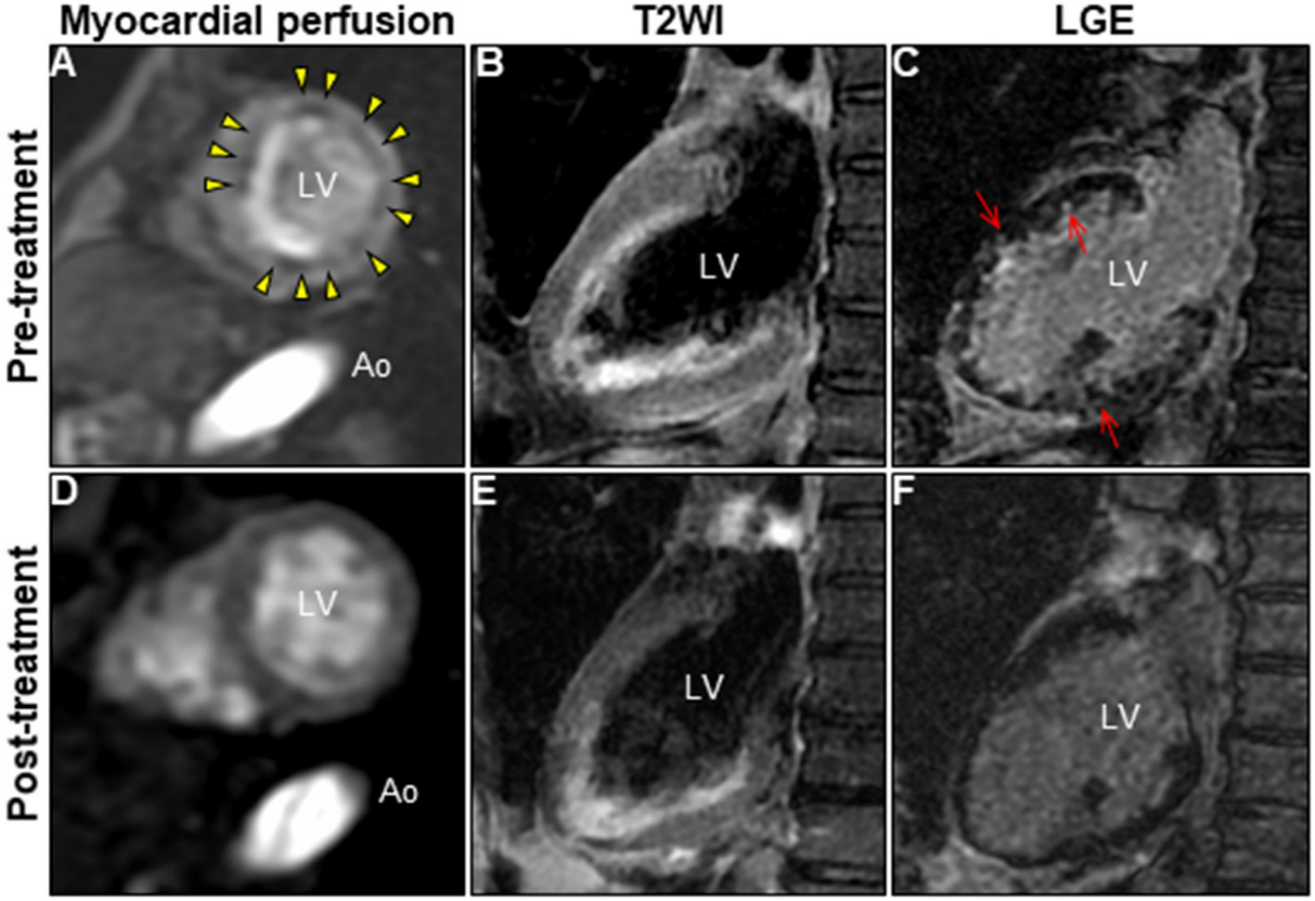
Changes in CMR findings in patients following corticosteroid treatment. CMR findings before (A–C) and after corticosteroid treatment (D–F). Myocardial first-pass perfusion imaging at rest (A,D), T2WI of the 2-chamber view (B,E), and LGE image (C,F). Ao, aorta; CMR, cardiac magnetic resonance; LGE, late gadolinium enhancement; LV, left ventricle; T2WI, T2-weighted image.
